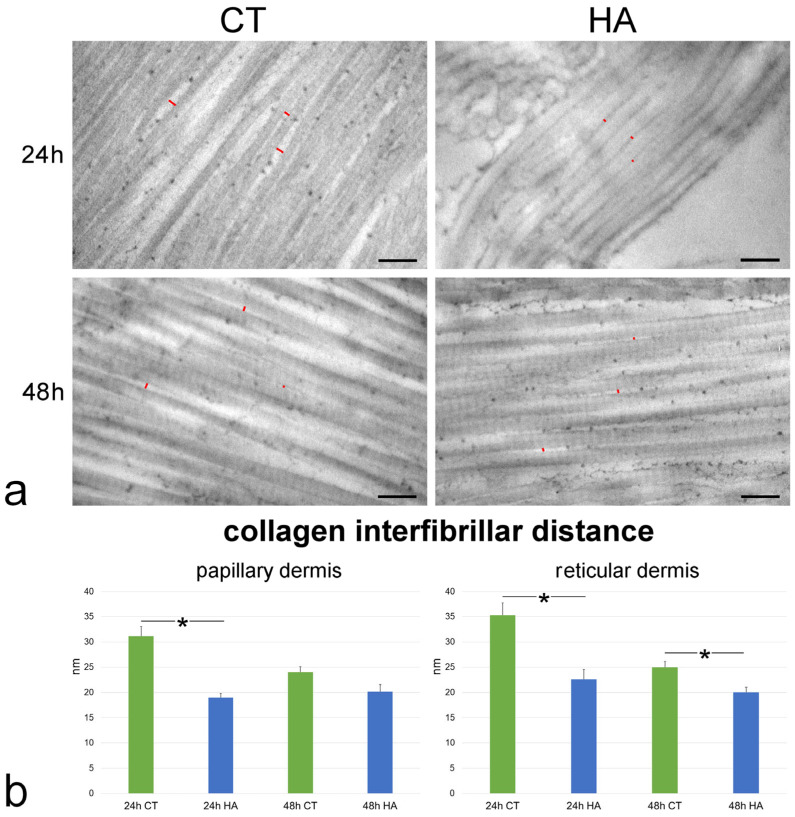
(a) TEM images showing collagen fibrils in the reticular dermis of control (CT) and hyaluronic acid (HA)-treated skin. Red lines indicate some interfibrillar distances. Bars, 200 nm. (b) Mean ± SE values (*n* = 40) of collagen interfibrillar distance in papillary and reticular dermis of control (CT) and hyaluronic acid-treated (HA) skin samples at 24 and 48 h. The asterisks (*) indicate a statistically significant difference between HA-treated samples and the relative CT.
